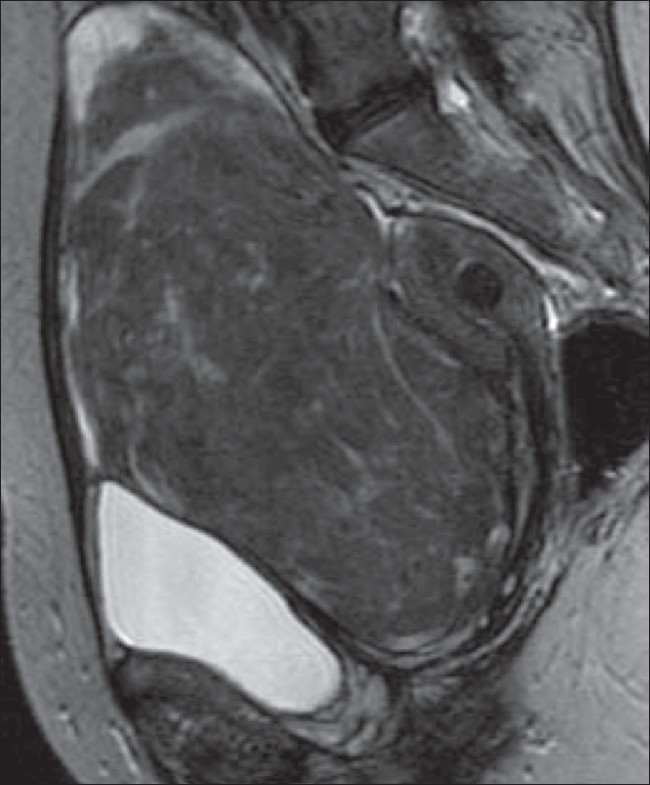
A 59-year-old woman with an abdominal mass and discomfort. Sagittal T2W MRI image shows a 15-cm pedunculated, subserosal fibroid arising from the anterior uterus. There is also a small intramural fibroid lying posteriorly
